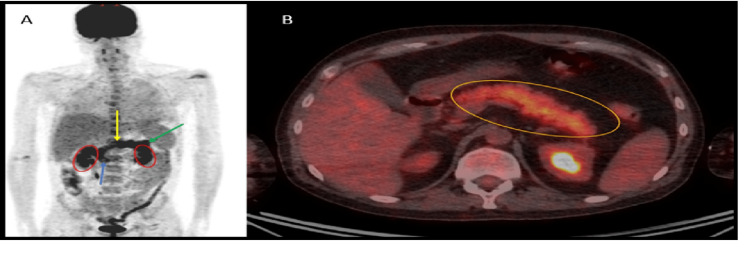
A. PET-CT planar image showing diffuse uptake in the pancreas, which is projected between the kidneys (red ovals). The blue arrow shows the pancreas head, the yellow arrow the pancreas body, and the green arrow the pancreas tail. B. Fused axial PET-CT showing the pancreas outlined by the orange oval, with diffuse FDG uptake within the pancreatic parenchyma PET: positron emission tomography; FDG: 18-fluoro-2-deoxyglucose
